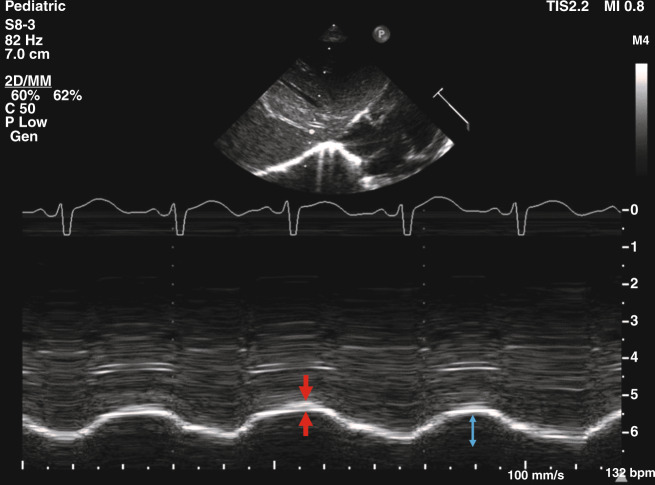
Diaphragmatic ultrasound Sonographic image showing M-mode measurement of diaphragmatic thickness (between the two arrows) and excursion of the right hemidiaphragm (bidirectional arrow) in a healthy term infant.
